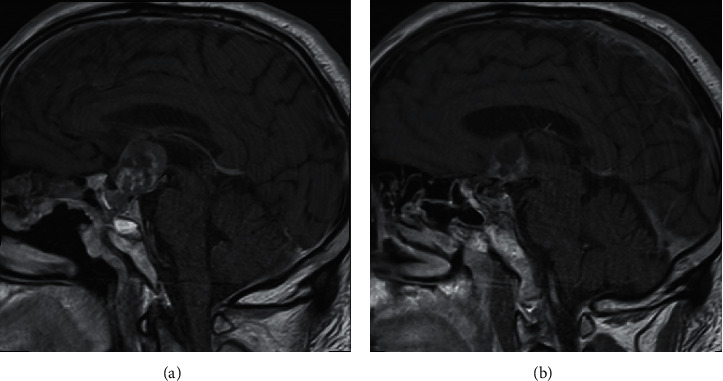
Initial MRI without gadolinium ordered by primary care provider, revealing a suprasellar mass extending into the anterior portion of the third ventricle and displacing the hypothalamus. There is evidence of a robust septal flap and residual fat graft from prior resection.
